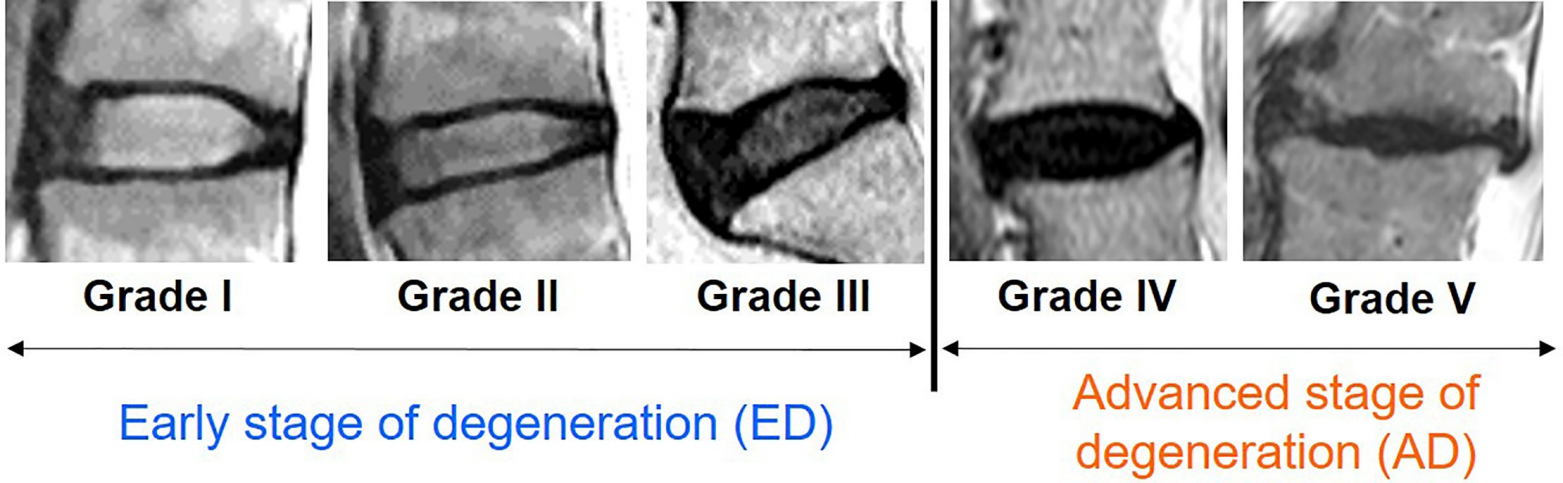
Magnetic resonance imaging (MRI) of human intervertebral disc classified according to the Pfirrmann grading system[22]. Grades I to III were classified as early stage degeneration (ED). Grade IV and V were classified as advanced stage degeneration (AD).
